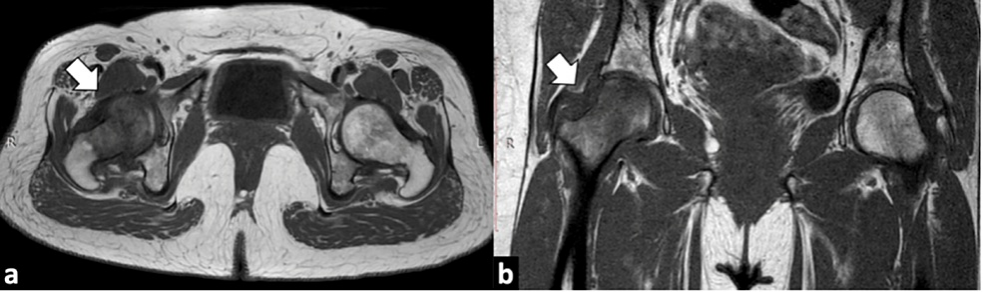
MRI hip images. Axial T1W image (a) and coronal T1W image (b) showing hypointense signals right femoral head and neck.
